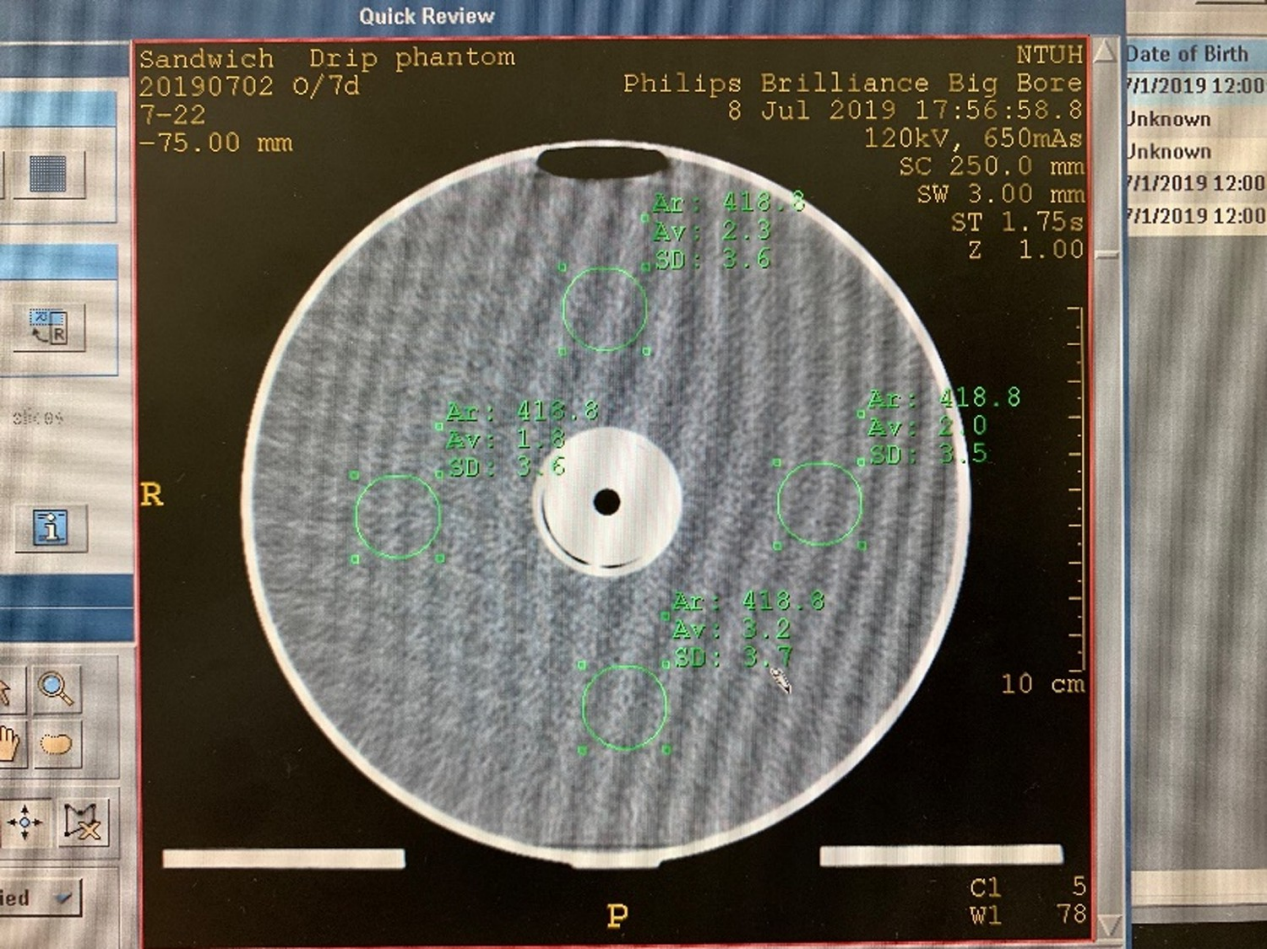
CT image of the water-filled uniformity slab.
